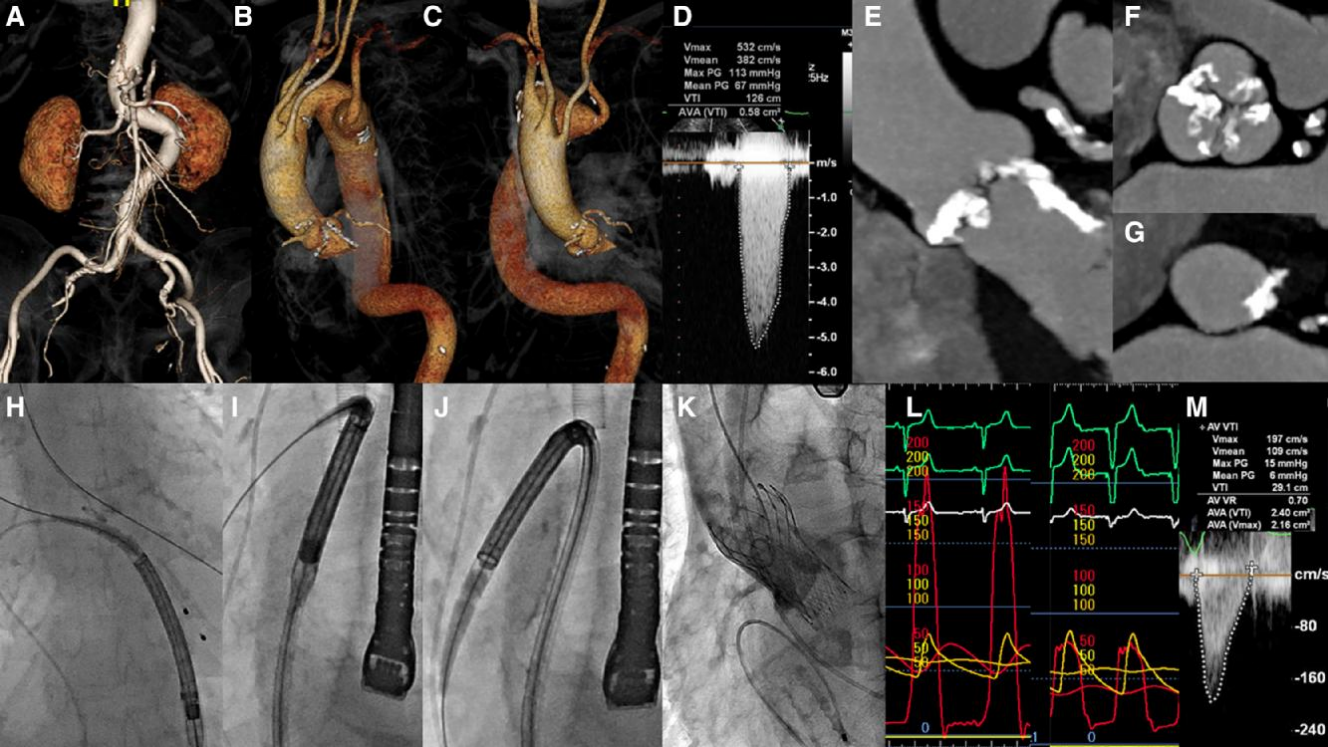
Tortuosity of abdominal aorta (*A*), aberrant left subclavian artery and right subclavian artery. Kommerell diverticulum with a right-sided aortic arch (*B*), a strong angulation and from the descending to the abdominal aorta was sharply curved (*C*), echocardiographic finding confirmed severe aortic stenosis (*D*), baseline computed tomography angiography demonstrated an excessive calcium proliferation was evident from the annulus to the left ventricle outflow excessive calcification (*E* and *F*), annular area of 357 mm^2^, an annular perimeter of 66.7 mm (*G*), the 14 French-compatible InLine sheath successfully passed through the abdominal aorta with the support of a Confida stiff guidewire (*H*), the Evolut-FX successfully transversed the sharply curved descending aorta and the severely angulated Kommerell diverticulum with a right-sided aortic arch (*I* and *J*), the Evolut-FX was successfully implanted (*K*), resulting in an improved post-operative haemodynamics (*L*) and echocardiogram showing a mean gradient of 6 mmHg (*M*).
